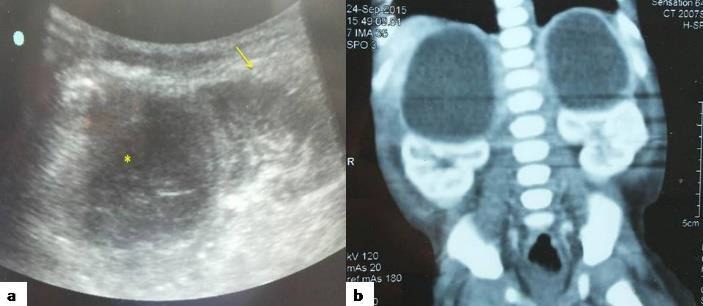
Case 1, a. Abdominal USG showing supra renal hypoechoic mass with internal echoes and debris (asterix) with inferior displacement of kidney (arrow) b. Abdominal computed tomography image (coronal section) showing well-circumscribed, thin-walled, peripheral-enhancing cystic lesions in bilateral suprarenal areas with inferior displacement of bilateral kidneys.
